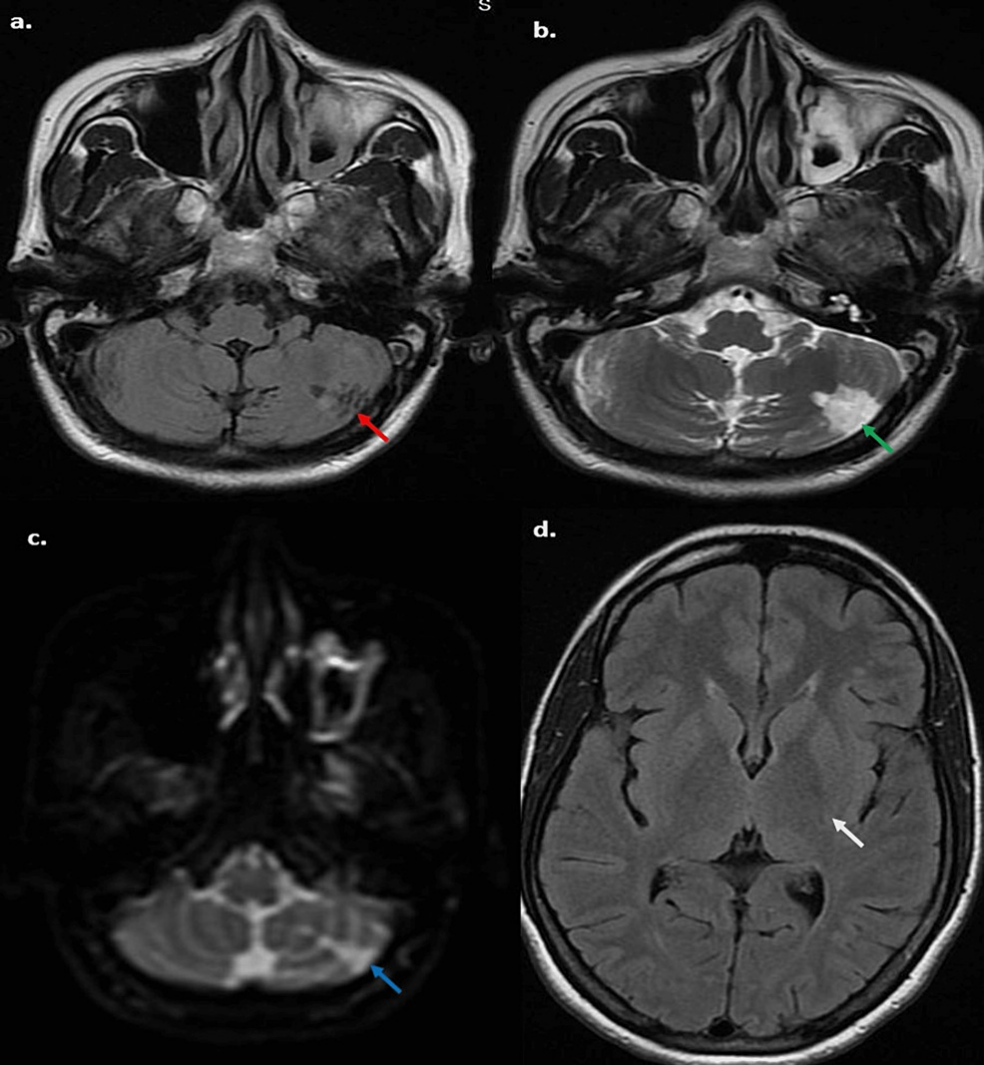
MRI of the brain (a) FLAIR axial sequence showing hypointensity in the left cerebellar hemisphere(red arrow), (b) T2 axial sequence showing hyperintensity in the left cerebellar hemisphere(green arrow), (c) MRI diffusion sequence showing diffusion restriction in the same region (blue arrow) with overall features suggesting infarction in the left cerebellar hemisphere. (d) MRI brain showing normal supratentorial structures (white arrow). MRI: magnetic resonance imaging, FLAIR: fluid-attenuated inversion recovery.
